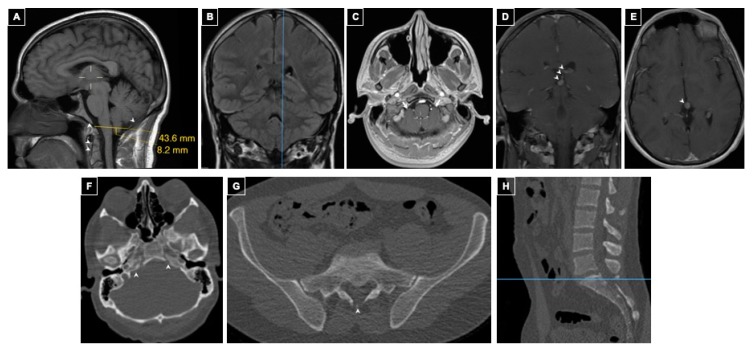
Patient 2 Intracranial and Spinal Imaging. Panels (A–E)*:* MRI of the brain. Panel (A): Sagittal post-contrast T1-weighted sequence shows the left cerebellar tonsil at the lowest tonsillar position, 8 mm through the foramen magnum. Narrow, underdeveloped clivus and occipital bone as well as uncalcified synchondroses of the odontoid are also seen (arrowheads). Panel (B): Coronal T1-weighted sequence demonstrating the location of the measurement in A. Panel (C): Axial post-contrast T1-weighted sequence showing crowding of the brainstem by the cerebellar tonsils in the foramen magnum (arrows). Panel (D): Coronal T1-weighted venous phase post-contrast sequence shows a developmental venous anomaly of the Vein of Galen confluens draining the choroid plexus through the velum interpositum (arrowheads). Panel (E): Axial T1-weighted venous phase post-contrast sequence showing the anomalous Vein of Galen confluens. Panel (F): Axial CT of the head showing petroclival dysraphism or uncalcified petroclival synchondrosis (arrowheads). Panel (G): Axial CT of lumbo-sacral spine shows occult dysraphism of S1 (arrowhead) and sacral segmentation anomalies (arrows). Panel (H): Sagittal lumbar CT demonstrating the level of the image shown in G.
